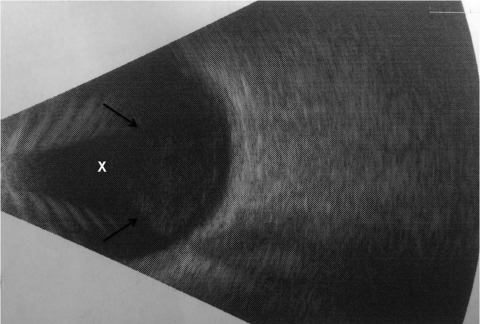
Ocular ultrasound demonstrating hyperechoic, punctate opacities (arrows) within the vitreous chamber (X) of a patient with coccidioidomycosis, California, USA.
